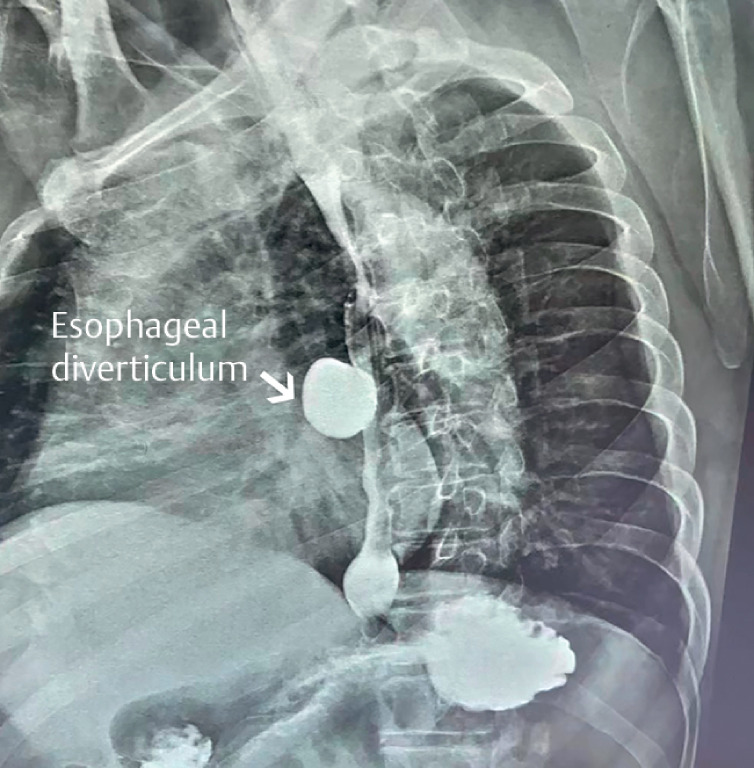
Preoperative upper gastrointestinal barium radiography showed a 3.0-cm mid-esophageal diverticulum.
